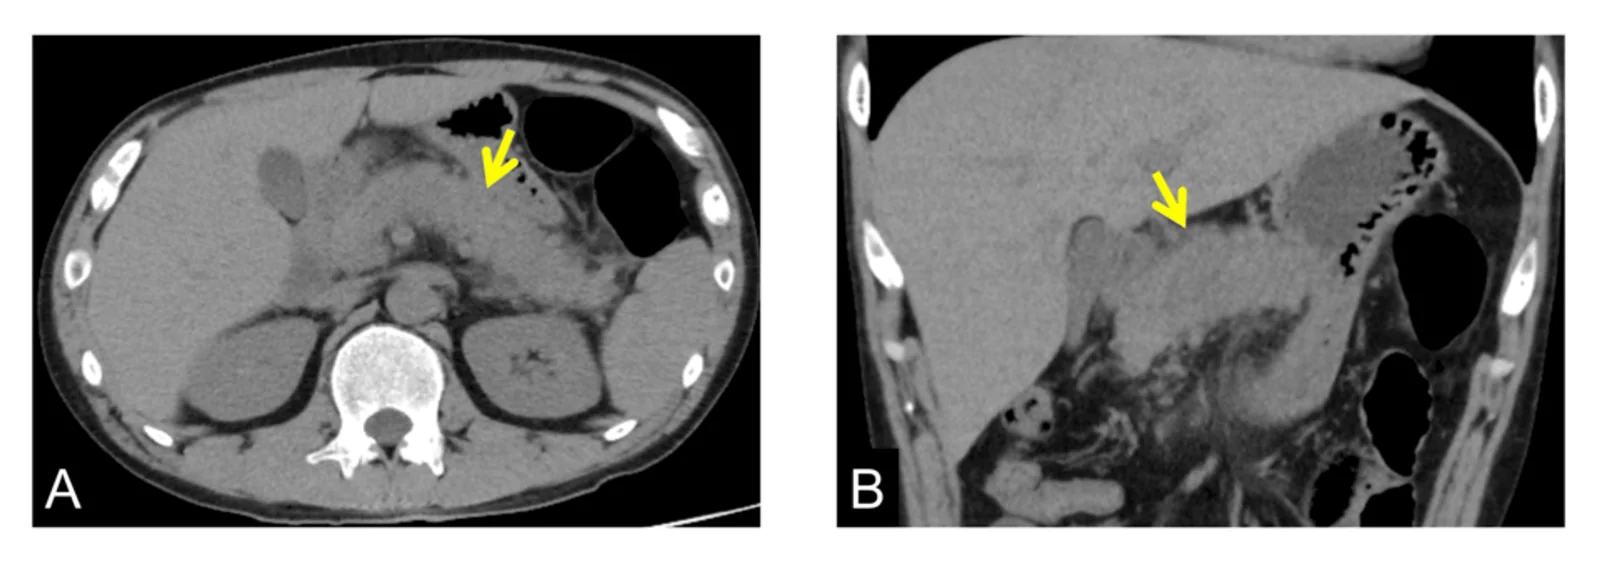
Noncontrast abdominal CT images obtained during the episode of acute pancreatitis in 2023. (A) Axial and (B) coronal views show no evidence of pancreatic calcification. The yellow arrow indicates the pancreas.
